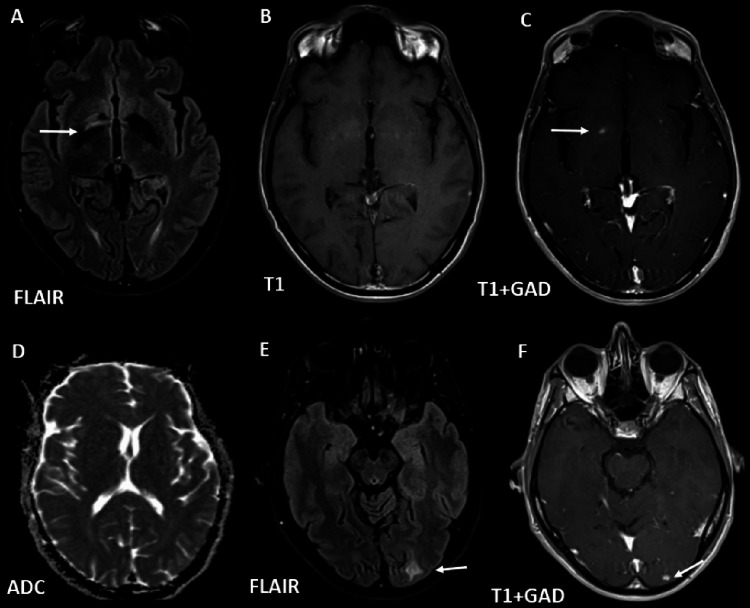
Brain magnetic resonance imaging Axial FLAIR image showing lesion with hypersignal in the right sublenticular region (A, arrow), not perceptible on T1-weighted images (B), demonstrating nodular enhancement after contrast (C, arrow), without diffusion restriction (D), FLAIR axial images (E, arrow) revealing a left occipital subcortical white matter lesion with post-contrast nodular enhancement (F, arrow). FLAIR: fluid-attenuated inversion recovery
